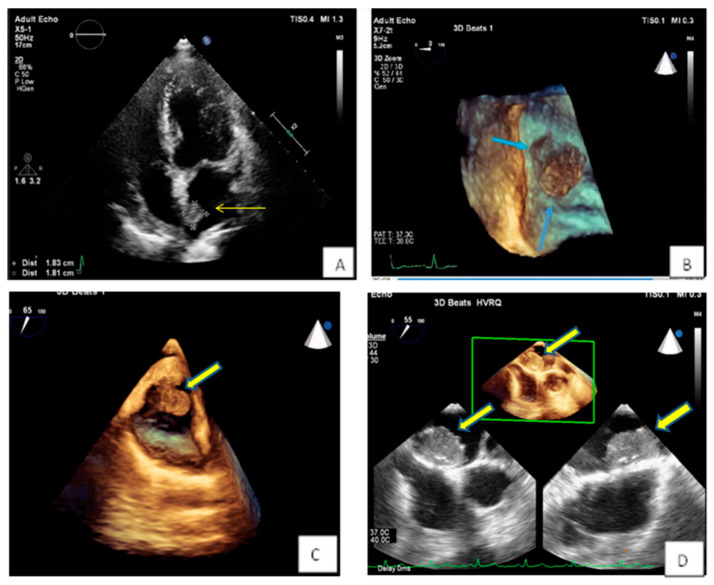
(A,B) Spherical lesion 1.8 × 1.8 cm attached through a wide base close to fossa ovalis was depicted at TTE (A) (yellow arrow) and subsequently TEE (B) (blue arrows—3D zoom). The location of the tumor in the left atrium as well as its morphological characteristics are compatible with a myxoma. (C) 3D TEE showing a polypoid (yellow arrow), with a smooth or mildly lobar surface located at the entrance of the inferior vena cava. Echocardiographic findings are consistent with a myxoma in a rare position. (D) 3D TEE depicting a large mass with spot calcific areas located at the fossa ovalis with a wide base, findings consistent with a left atrium myxoma. Please note the areas with higher echogenicity within the mass (yellow arrows).
